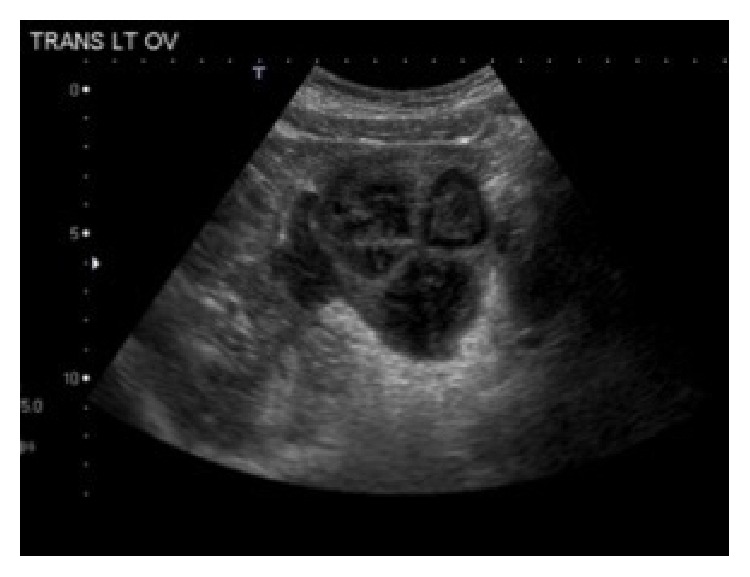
Ultrasound image showing large, complex cystic mass in the left adnexa measuring 5.9 × 8.4 × 5.5 cm in dimensions.
